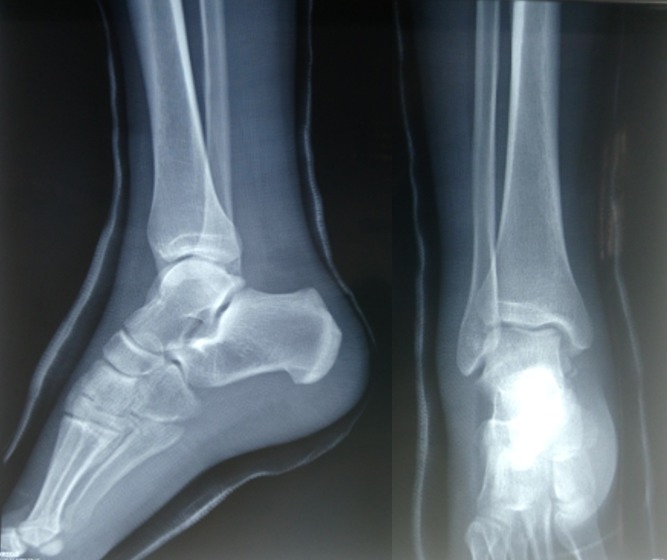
Radiographie standard de contrôle après réduction de la luxation
